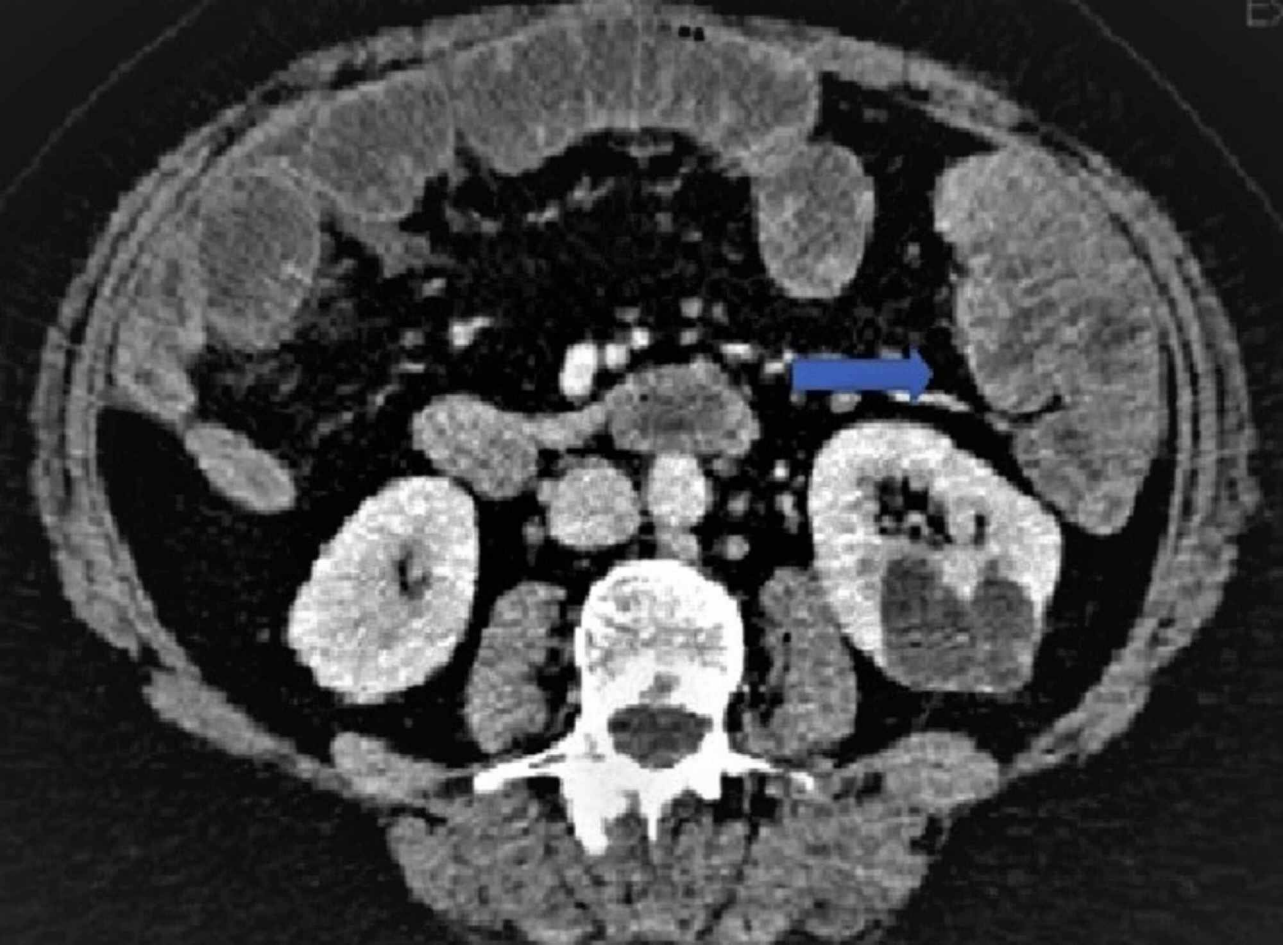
CT scan Large stool burden in the colon (shown by arrow) CT: computed tomography
